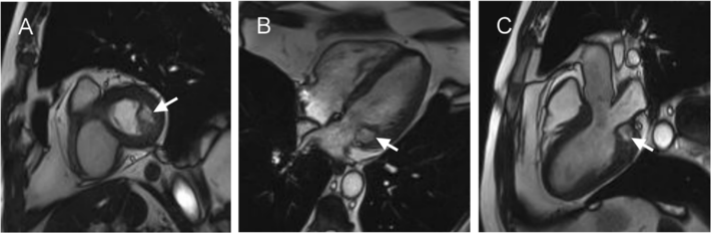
Diastolic steady-state free-precession MR images of the mass in short-axis (A), 4-chamber (B) and 3-chamber (C) orientations. The mass (arrows) appears in the left ventricle, with a hyperintense core compared to myocardium and isointense to blood, adherent to the posterior papillary muscle and thickened posterior mitral valve leaflet.
